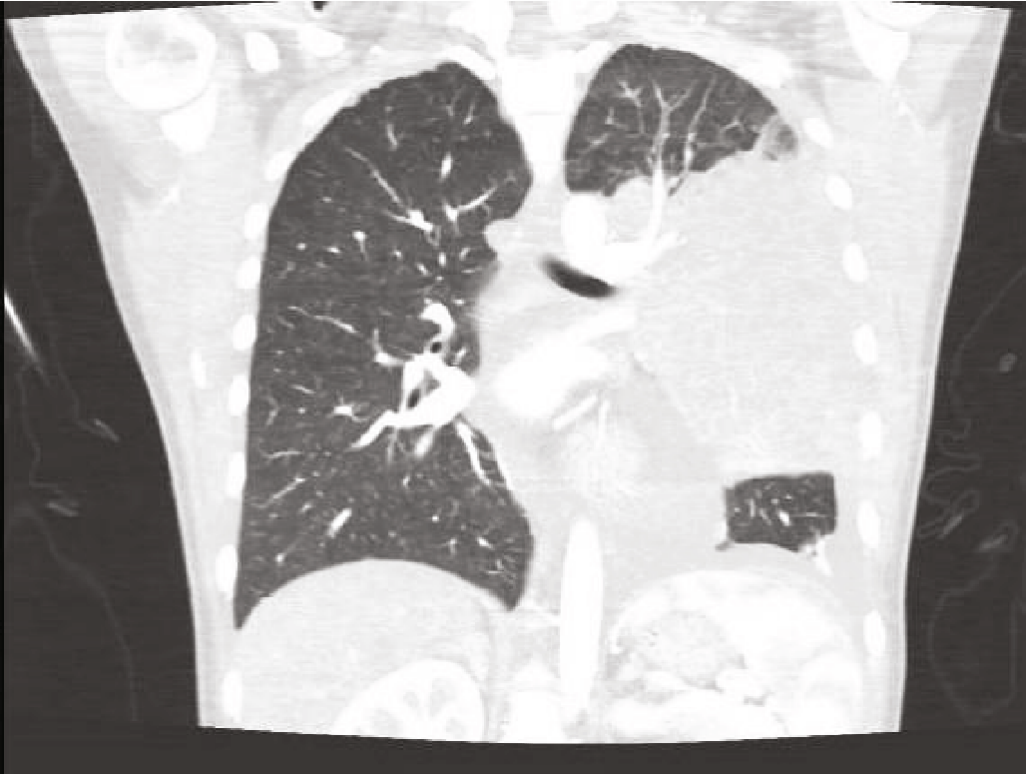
CT scan demonstrating a mediastinal mass narrowing the left mainstem bronchus and involving the pericardium and myocardium of the left ventricle.
